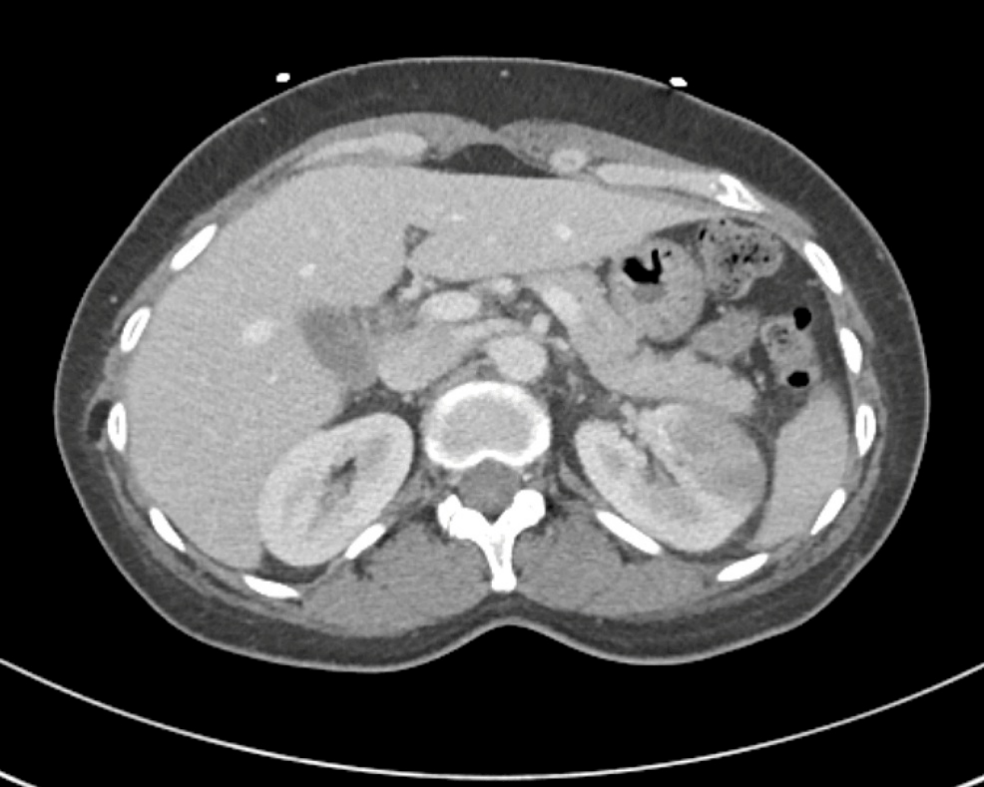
CT scan of abdomen and pelvis revealing areas of low-attenuation within the anterior lateral and posterior medial mid-left kidney suggestive of acute pyelonephritis
